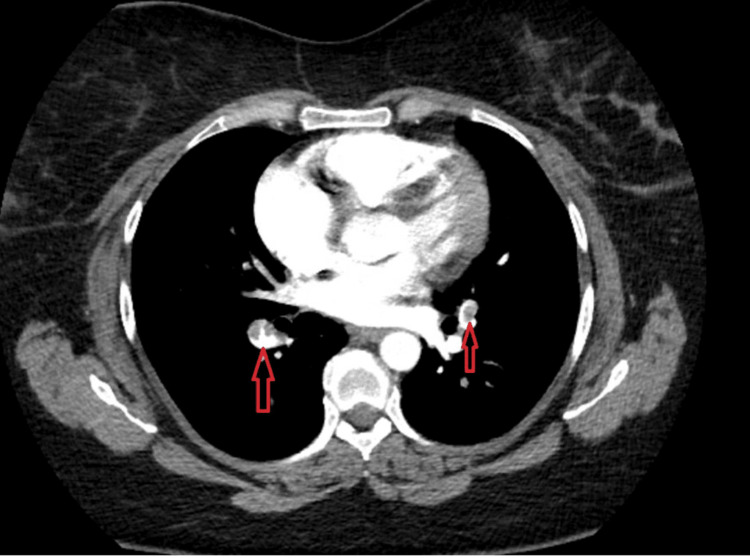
CT angiogram. Notable for bilateral segmental pulmonary emboli with (red arrows) evidence of right heart strain
